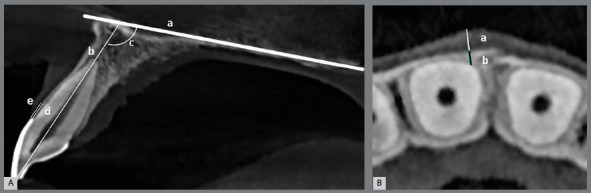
Cone-beam computed tomography images. A) Parasagittal image: (a) palatal line, between the anterior and posterior nasal spines; (b) long axis of the maxillary right central incisor; (c) tooth inclination in relation to the palatal line; (d) cementoenamel junction-alveolar crest distance; (e) gingival margin-alveolar crest distance. B) Axial image: bone (a) and gingival (b) thickness.
